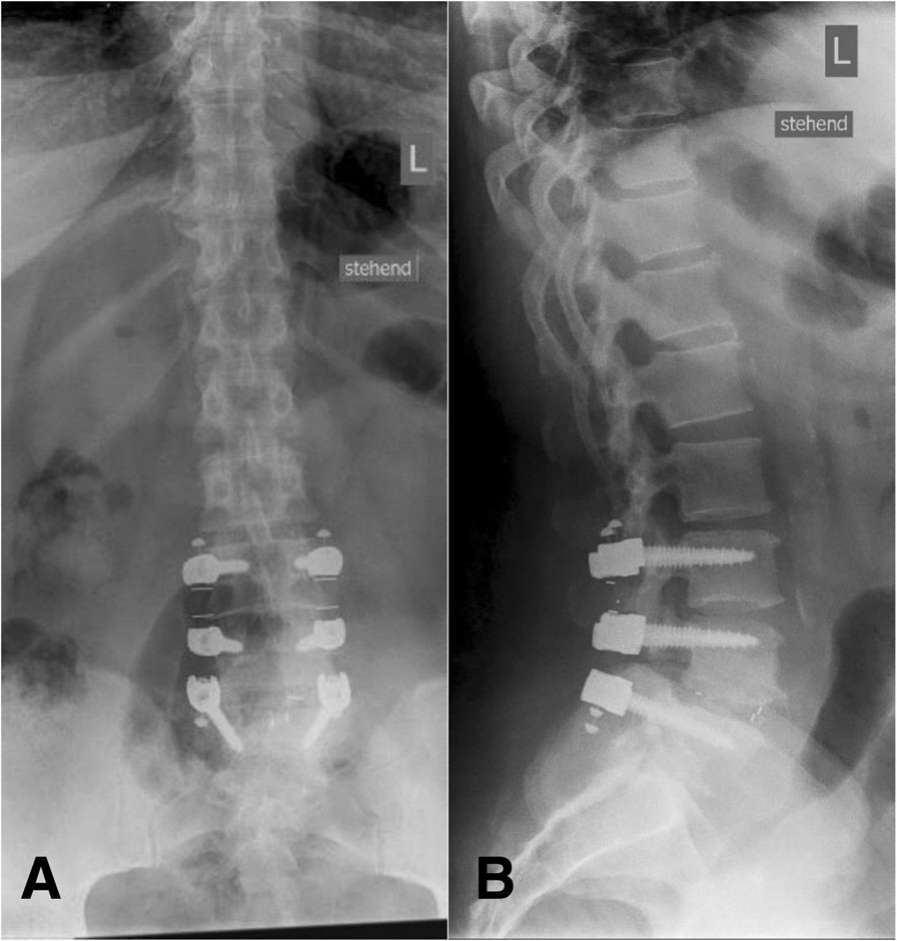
Lumbar spine anterior–posterior (a) and lateral (b) radiographs immediately after surgery
